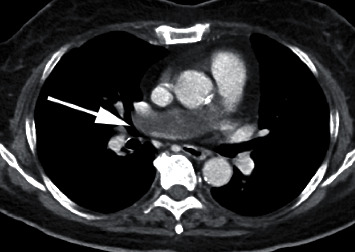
A contrast-enhanced computed tomography scan showing a tumor (arrow) in the right main pulmonary artery.
